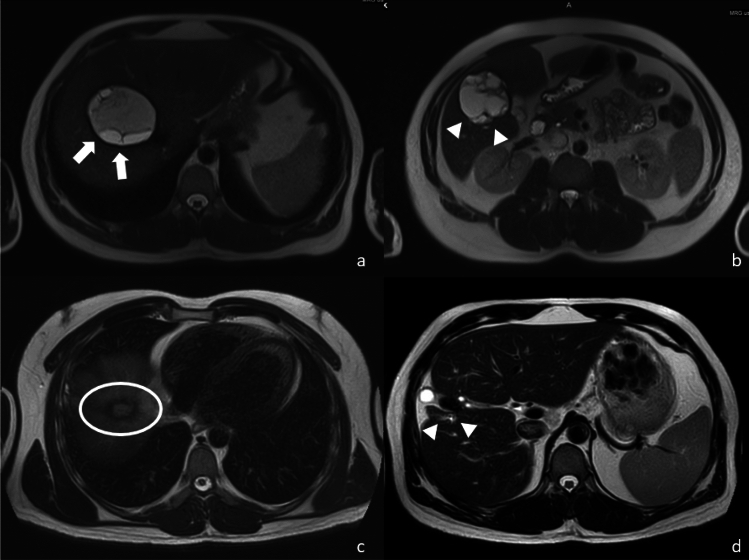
a A 34-year-old male patient who applied to the hospital presented with right upper quadrant pain, abdominal MRI revealed CE type 3b hydatid cysts located in segment 8 (a, white arrows) and segment 5 (b, white arrowheads) of liver. 18 months later, control MRI demonstrated that there was no active cyst or recurrence at previous cysts sites but some sequelae changes and calcifications (c, white circles; d; white arrowheads). b During the Mo-CAT procedure of the same patient, after inserting of Amplatz wire (a, white arrowheads), 12-French catheters (b-d, white arrows) were placed into the cysts. In cavitogram, there were lots of daughter vesicles (a and c, black arrowheads) within the cysts. In the first step, we destructed some daughter vesicles which located especially peripherally of the cyst that called “a liberated zone” (b, white circle). We continued the destruction of the daughter vesicles until there were no obvious daughter vesicles in the cavitograms (d)
